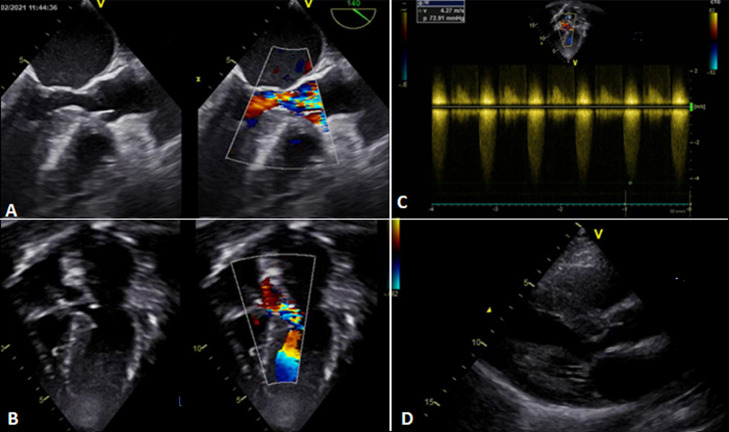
severe sub-aortic stenosis on echocardiogram with significant turbulence in the left ventricle outflow tract (A, B); and a peak instantaneous pressure gradient of 80 mmHg (C). Significant hypertrophy of the left ventricle as a consequence of the severe sub aortic stenosis (D)
